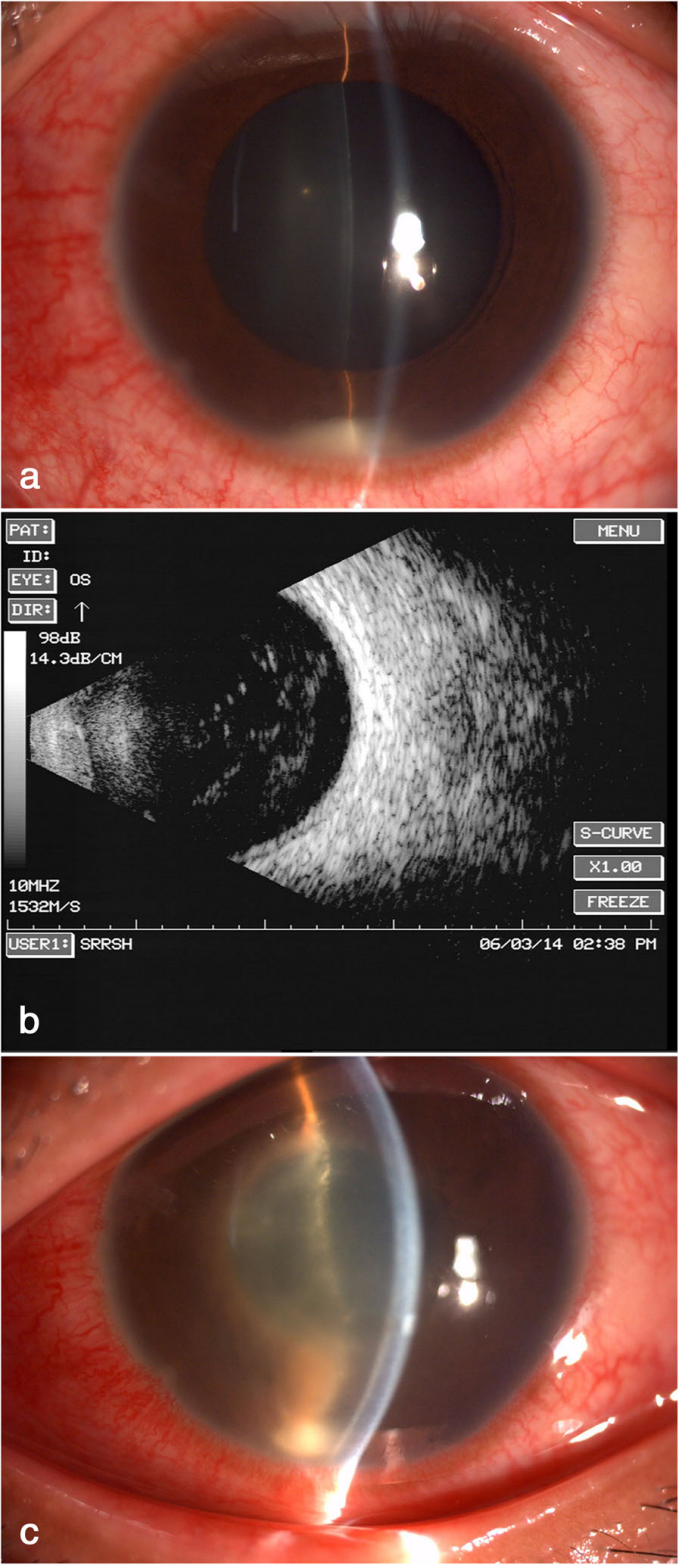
Pre-operative evaluation of the infected eye. a slitlamp examination at first presentation revealed moderate congestion in the left eye, with a transparent cornea, anterior cells4+, about two millimeters high hypopyon, a transparent lens. b ultrasonic B scan showed severe vitreous opacity without any posterior vitreous detachment. c after keeping stable for three days with vitreous injection of antibiotics, his left eye deteriorated obviously on the 4th day, with a vision of hand movement and an unobservable fundus
